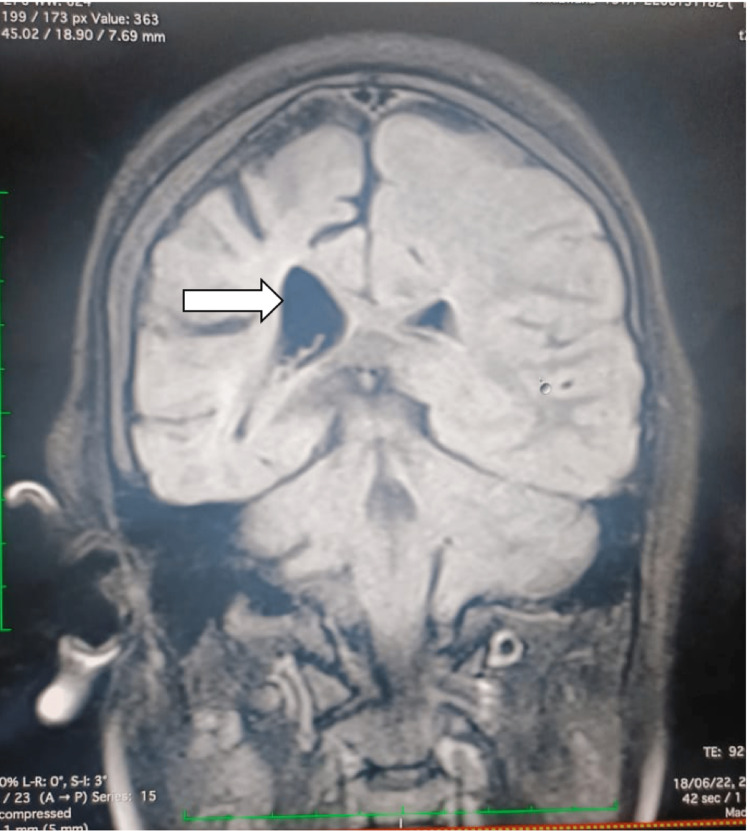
Coronal T2 FLAIR MRI of the brain demonstrates dilated lateral horn of right ventricle. MRI: magnetic resonance imaging; FLAIR: fluid-attenuated inversion recovery
